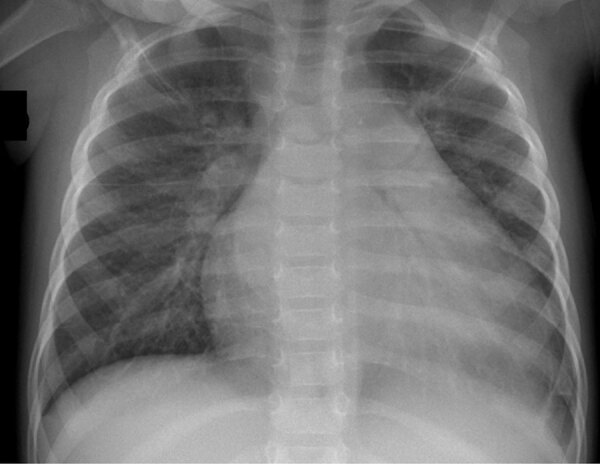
Chest radiograph of a pediatric patient with severe inflammatory cardiomyopathy caused by coxackievirus strain A9, northeastern France, 2024. Imaging was performed the day before admission to the pediatric ward and demonstrated unclear cardiomegaly.
